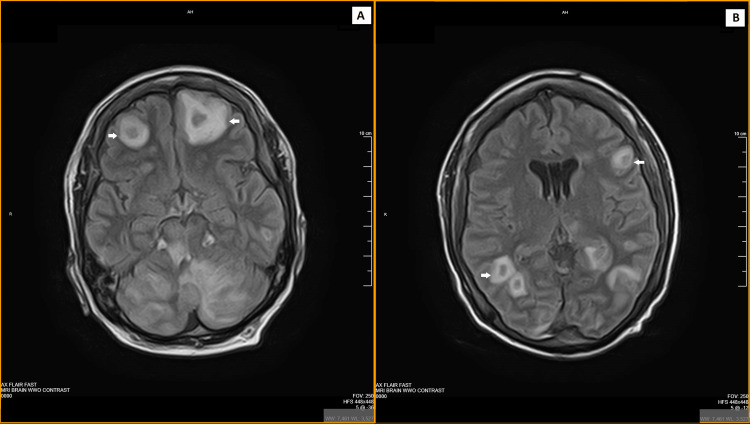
MRI brain on presentation with axial sections (flair) (both A and B) showing multiple ring-enhancing lesions (white arrows). MRI, magnetic resonance imaging
